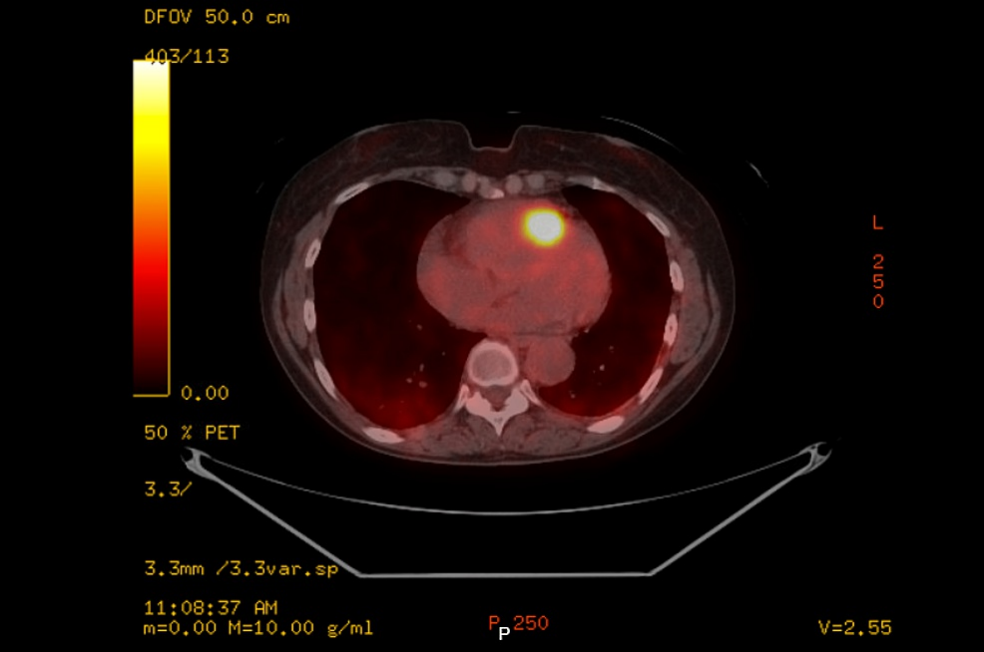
PET/CT scan PET/CT fused axial image showing increased fluorodeoxyglucose uptake by the RV mass. RV: right ventricle
